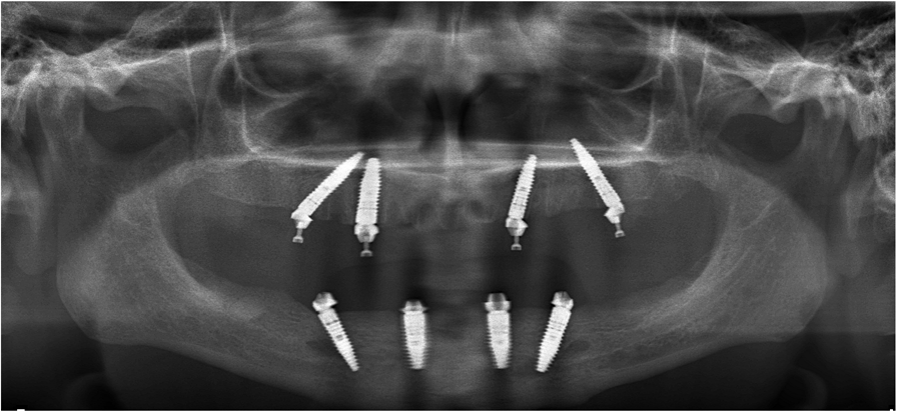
Panoramic X-ray after surgery
